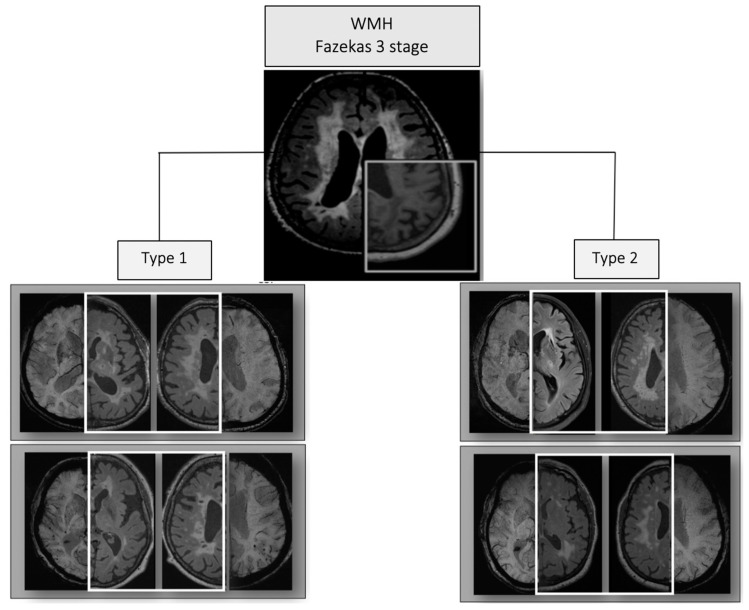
Characteristic changes observed in MRI types 1 and 2 of Fazekas stage 3 cerebral small vessel disease (cSVD) on FLAIR (highlighted with a white square) and axial susceptibility weighted imaging sequence (SWI) MRI slices.
